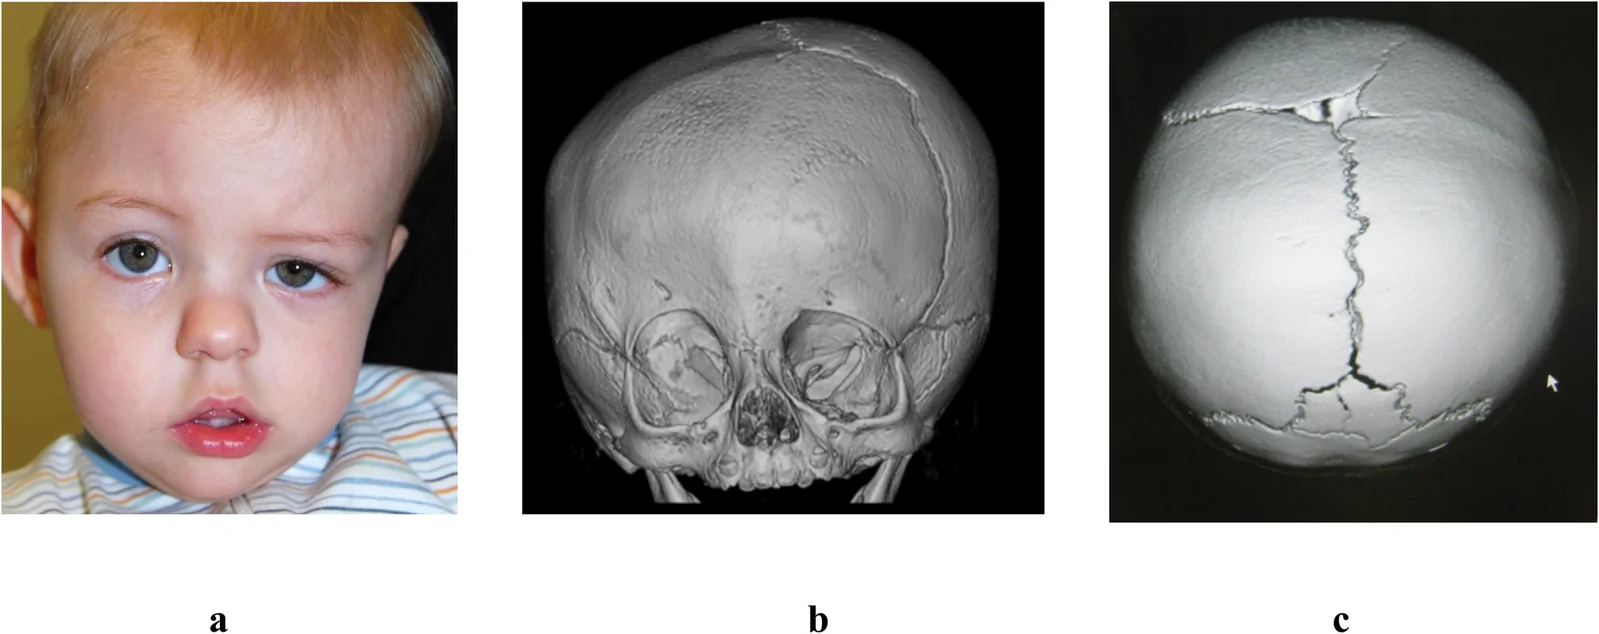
Non-Syndromic craniosynostosis patient with. a right superolateral brow elevation and orbital dystopia, b CT scan demonstrating retrusion of fright frontal bone and superolateral elevation of the right orbital rim, c right coronal suture synostosis. *With permission from Children’s Hospital of Philadelphia (CHOP)*.
